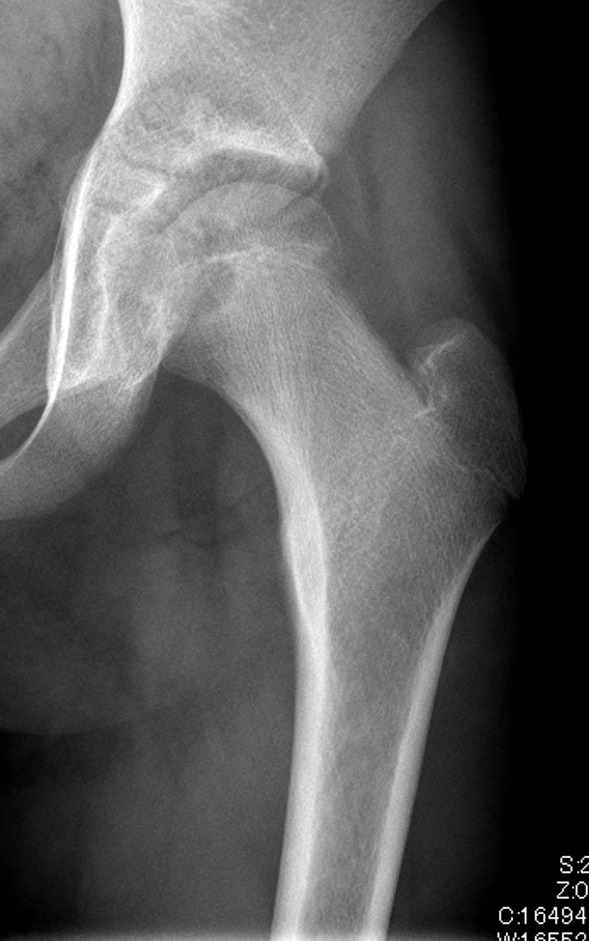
Preoperative X-ray images: Case 2. X-ray image showing a 1 cm × 1.5 cm osteolytic lesion with a marginal sclerotic rim
